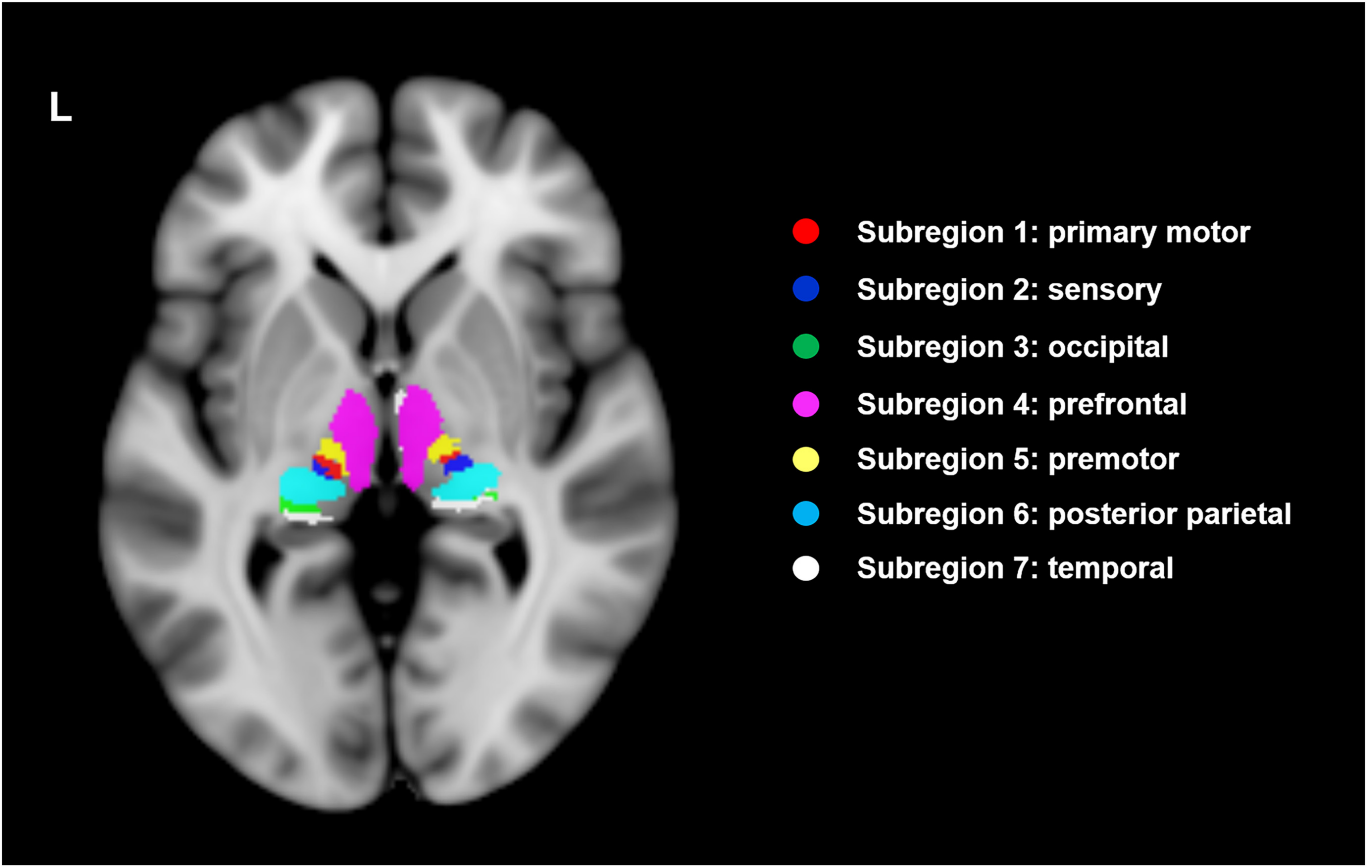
Thalamic subregions. Representative axial magnetic resonance imaging (MRI) of the thalamus subregions. A 25% threshold was used to binarize the original maximum probabilistic atlas publicly released in the FSL toolbox.
